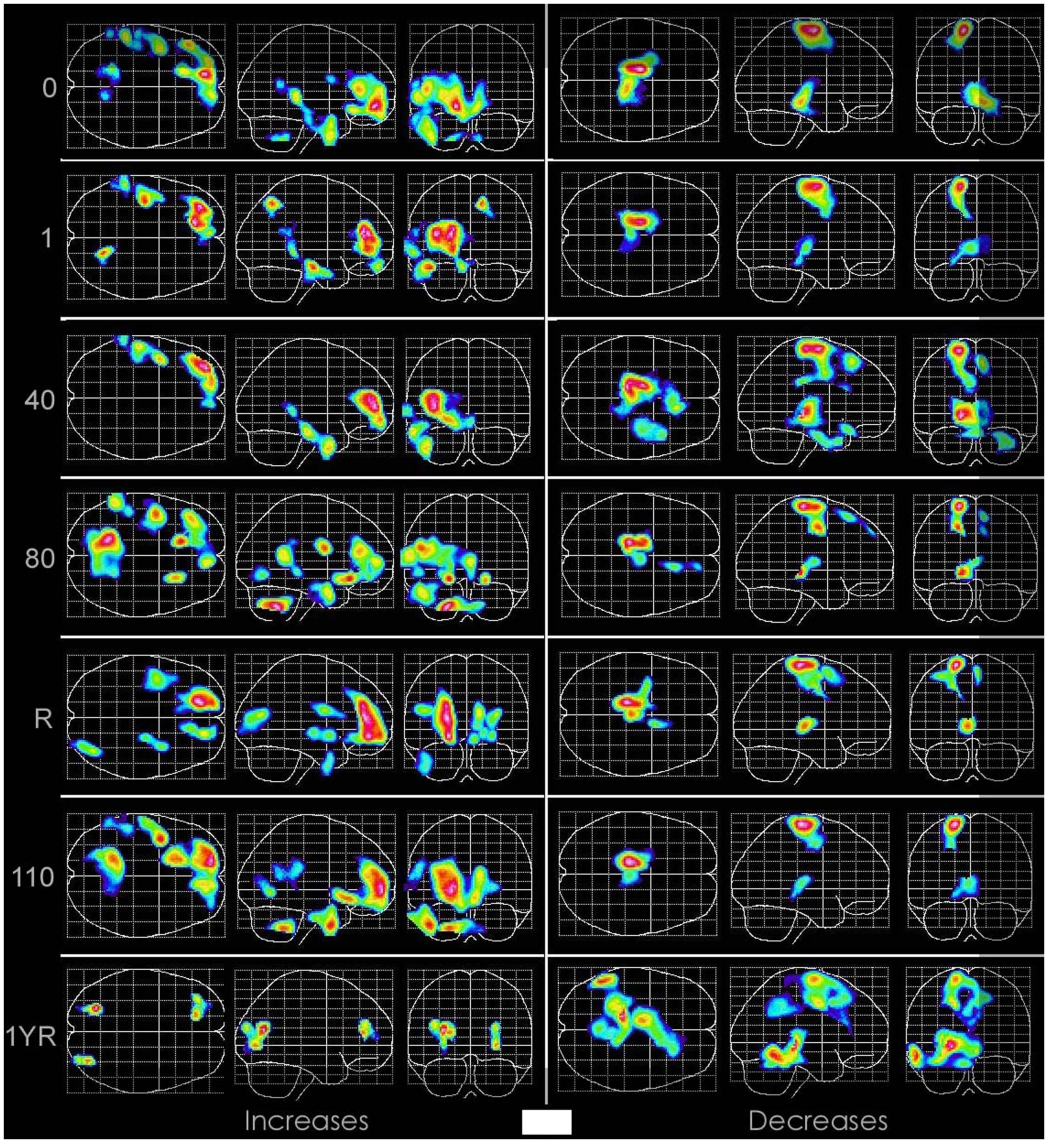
Sequential SPECT brain imaging of Subject #16 before the first HBOT (Row 0) then after 1, 40, 80 HBOTs, a 6-month break (Row R), 110 HBOTs, and 1 year post 110th HBOT (1 YR). Images are statistical parametric maps of selective coregistered slices. Color scheme: black indicates rCBF is within reference normal range. Increasing Z-scores (blood flow) are blue, green, orange, red, white on the left 3 columns and decreasing Z-scores with the same color scheme on the right 3 columns. SPECT at time 0 shows multiple areas of abnormally increased brain blood flow (top row left 3 images) and abnormally decreased brain blood flow (top row right 3 images) compared to normal and the other 6 patients in the study. Note intensification and broadening of Row R increased right frontal blood flow (6 months after 80th HBOT) that shows persistence and increases in the right frontal, posterior fronto-parietal and parietal, opposite anterior frontal, right temporal, and cerebellar areas after removal from the study for behavioral deterioration (Row 110), nearly all of which recede 1 year post study (1YR) to less than pre-HBOT levels (Row 0). Simultaneously, at 1YR post HBOT the right 3 panels show broadening of the adjacent areas with significant decreases in blood flow compared to the pre-HBOT level. Reproduced with permission Best Publishing Company (43).
